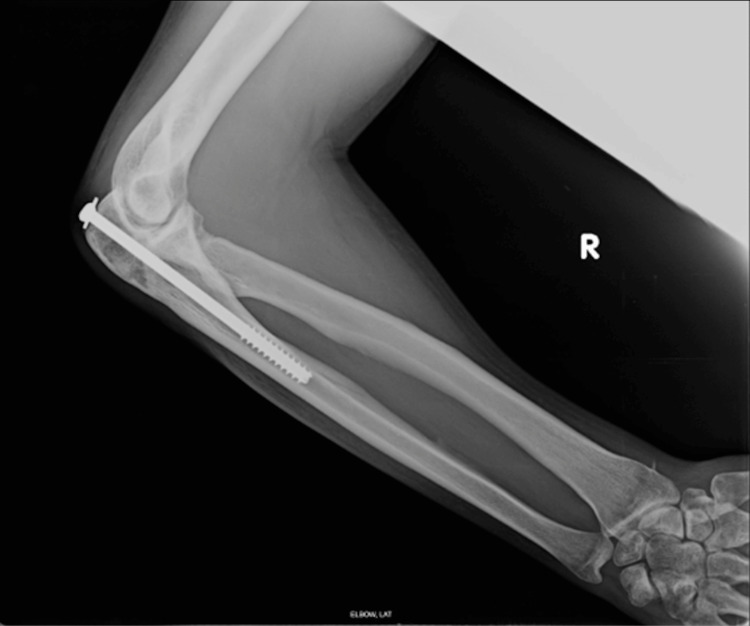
Right lateral elbow X-ray demonstrating retained olecranon screw
